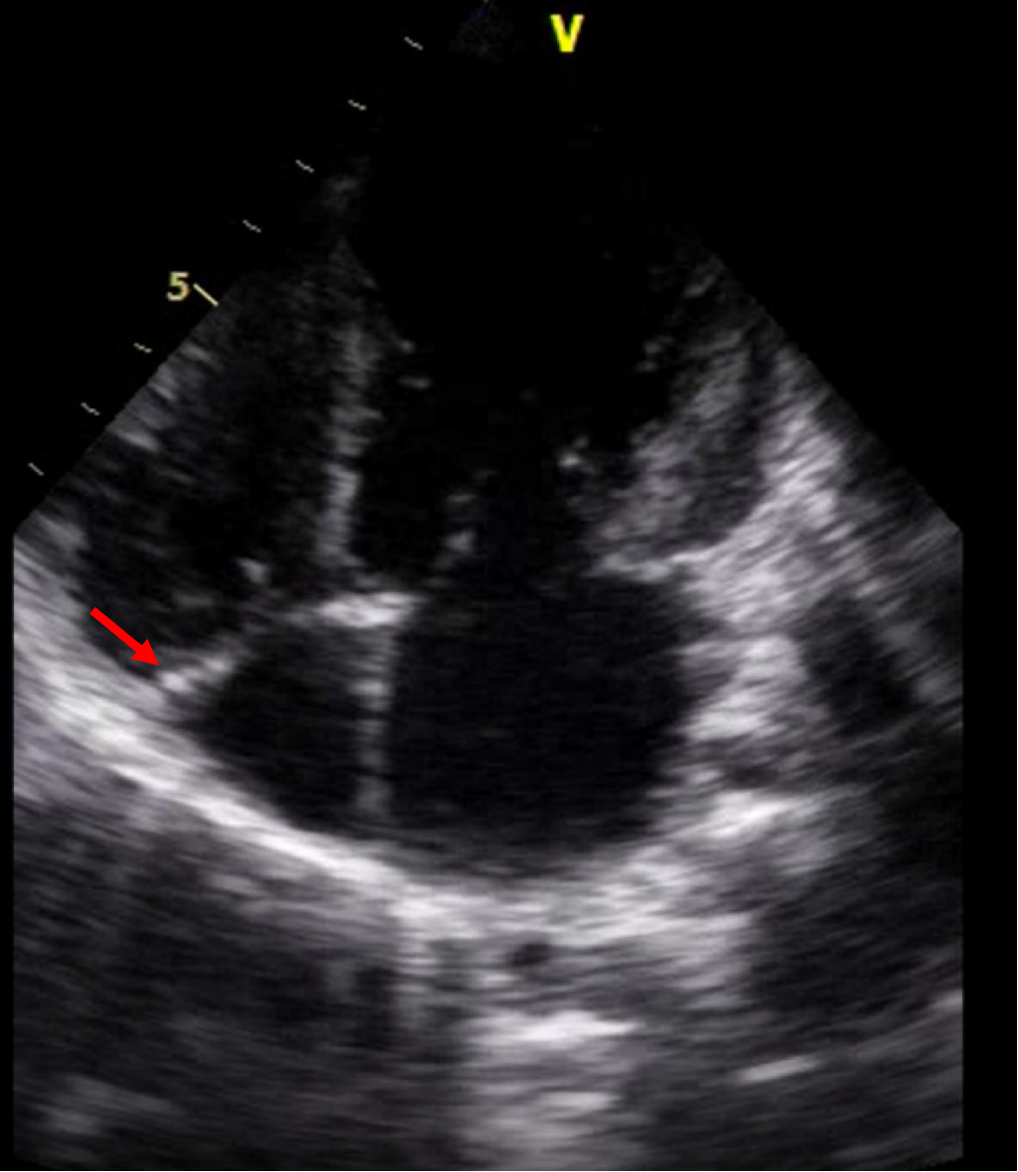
Apical four-chambers view of a transthoracic echocardiogram showing the membrane partitioning the right atrium (arrow)
